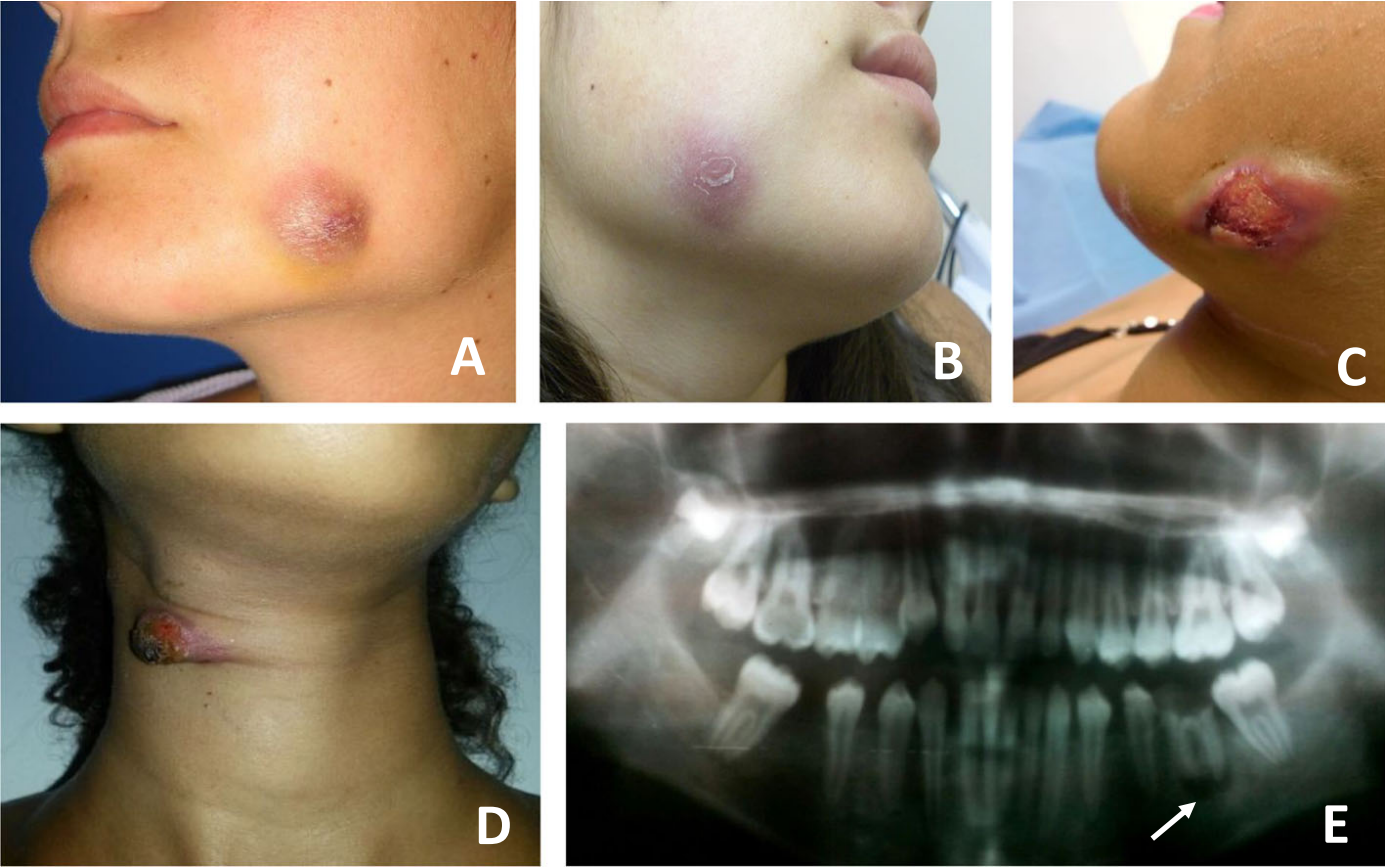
a-e The three patients from Caracas, Venezuela visiting our clinic for a diagnosis. a and b Patient 1 and patient 2 with the diagnosis of a dental sinus tract caused by respectively a *M. fortuitum* and *M. abscessus* infection. c and d Patient 3 with an infection due to *M. peregrinum*. Shown are the cutaneous facial sinus tract and an affected lymph node draining in the neck. The affected lymph node in the neck was removed with surgery. e A panoramic radiography of the patient 3 showing a radiolucent lesion of the periapical area of a mandibular molar (white arrow)
